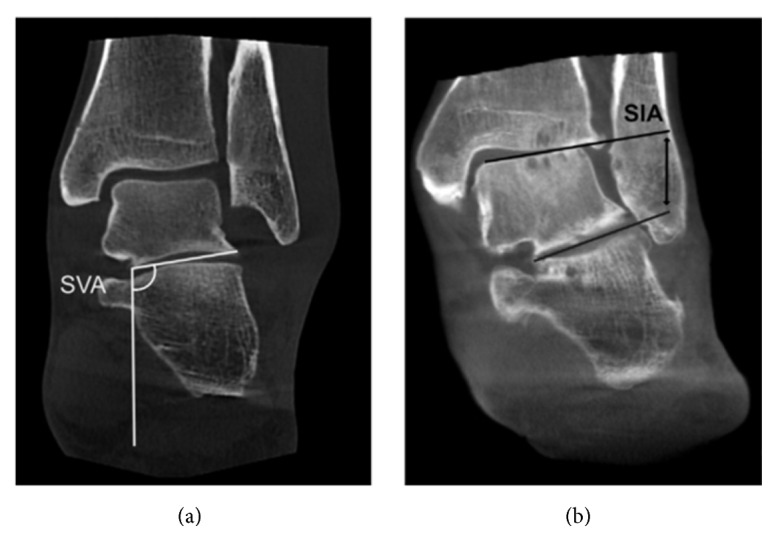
(a) Corresponding weight-bearing computed tomography (CT) scan. The orientation of the subtalar joint to the ground was assessed using the subtalar vertical angle (SVA). (b) Corresponding weight-bearing CT scan. The subtalar inclination angle (SIA) was used to assess the inclination of the subtalar joint. Figure 4 is reproduced from Krahenbuhl et al. 2017 [9].
